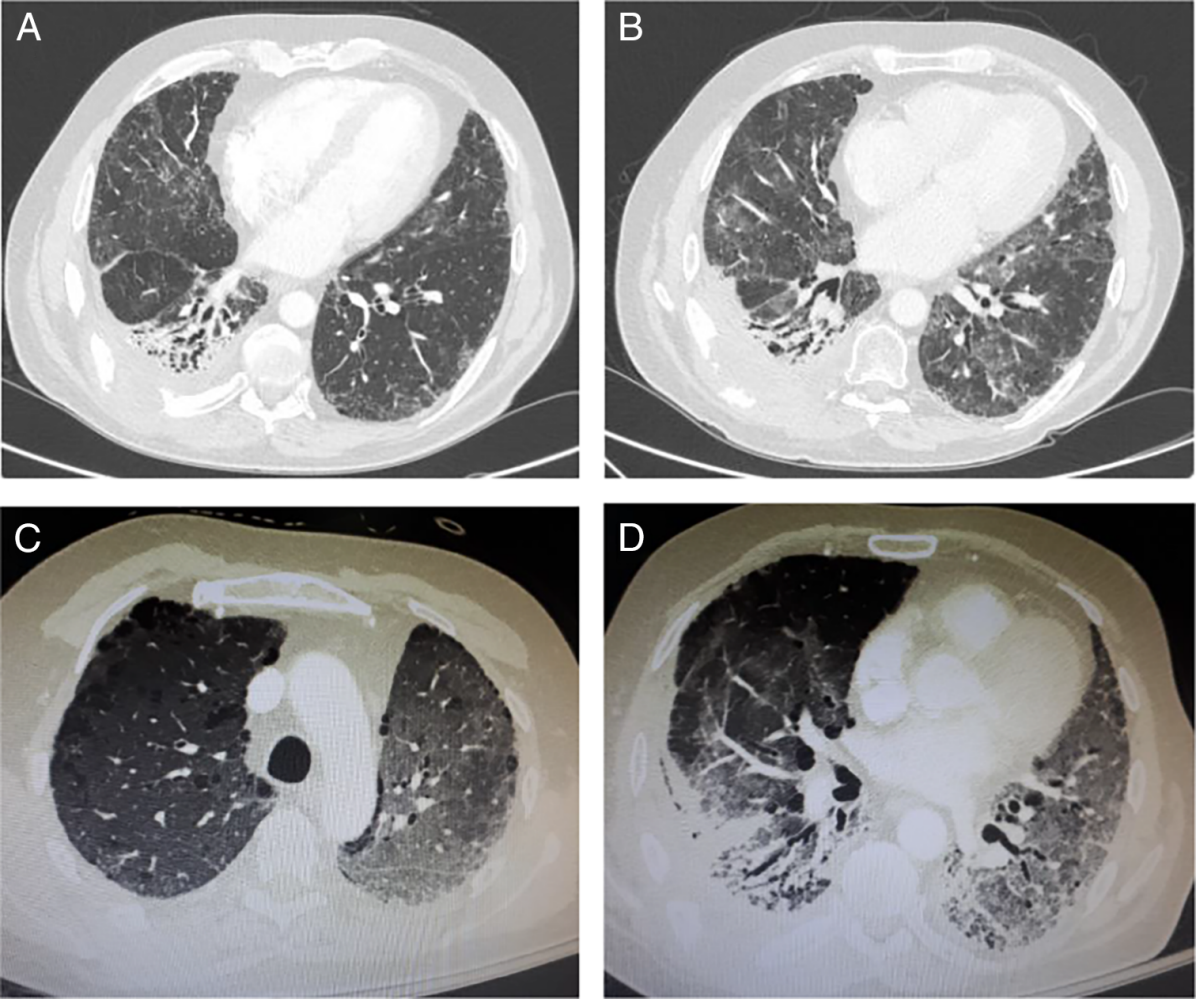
Computed tomography (CT) scan sequential imaging of primary squamous cell carcinoma of the pleura. (A, B) Evidence of pulmonary fibrosis on the right lower lobe due to radiation treatment, (A) one month and (B) seven months after radiation treatment. (C, D) Ground‐glass opacities all over the lung—lung toxicity due to nivolumab treatment.
